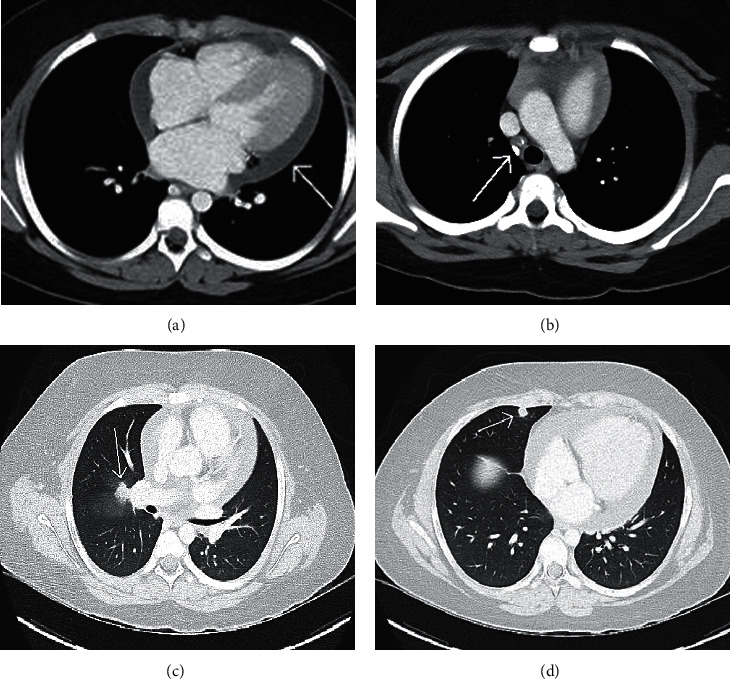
Axial CT images with contrast showing (a) large pericardial effusion and (b) calcified, but not enlarged right lower paratracheal region mediastinal nodes. (c) A 10 mm nodule at the right hilar region that has a mild spiculated appearance with adjacent ground glass opacities suggestive of hilar adenopathy versus pulmonary nodule. (d) Additional pulmonary nodule.
